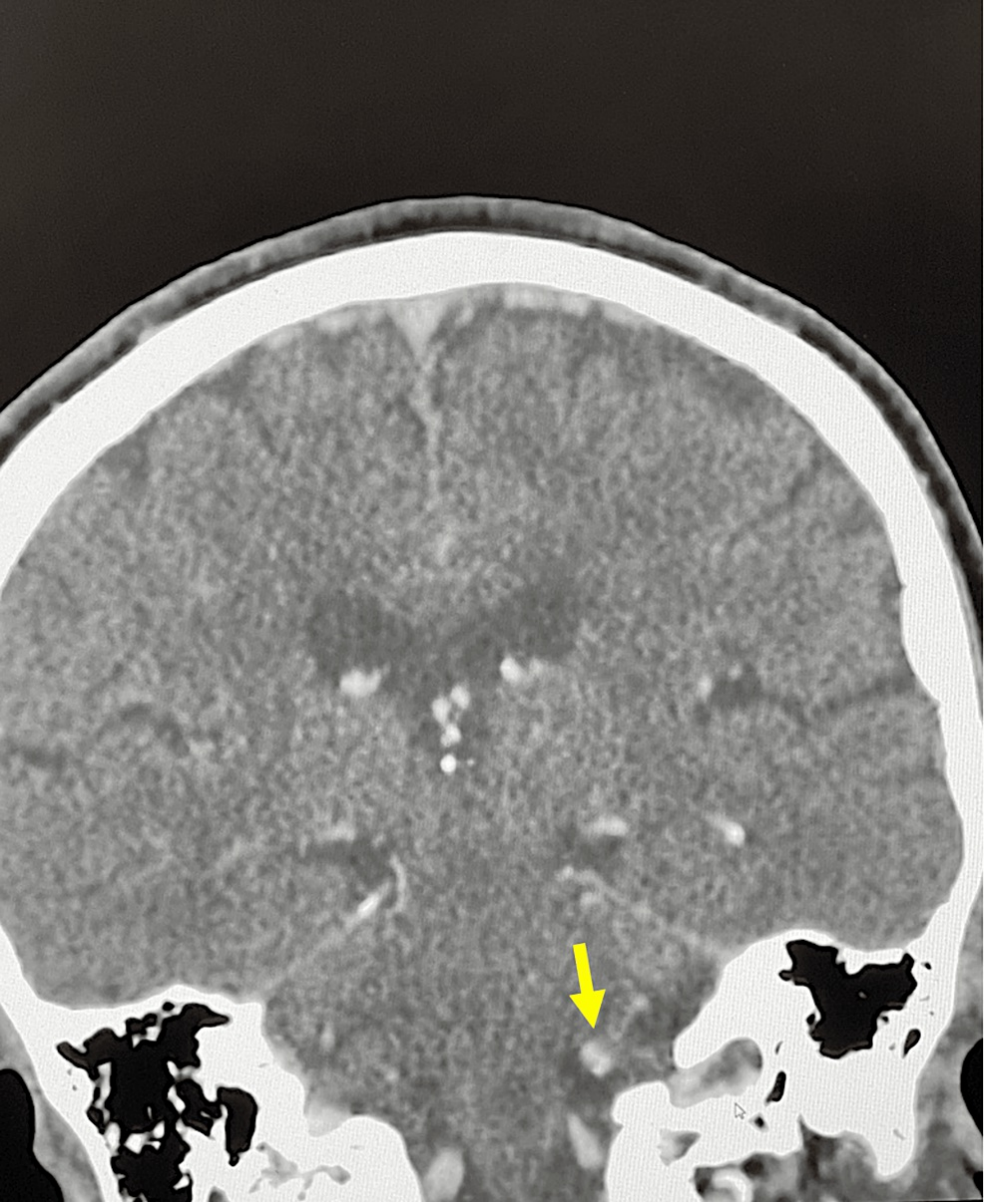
CT venogram demonstrating CVST with involvement of the left sigmoid sinus and left internal jugular vein. CT: computed tomography, CVST: cerebral venous sinus thrombosis
